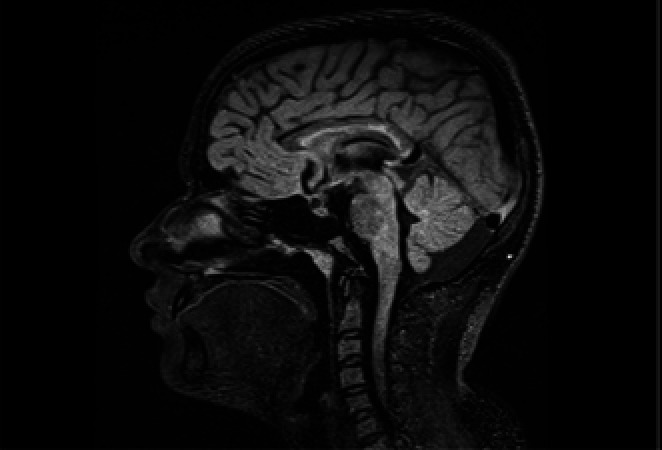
FIGURE 2 Right/Sagittal T2‐weighted MRI of the brain demonstrating widespread hypomyelination with subtle involvement of the cerebellar peduncles, sparing the cerebellar tonsils.
